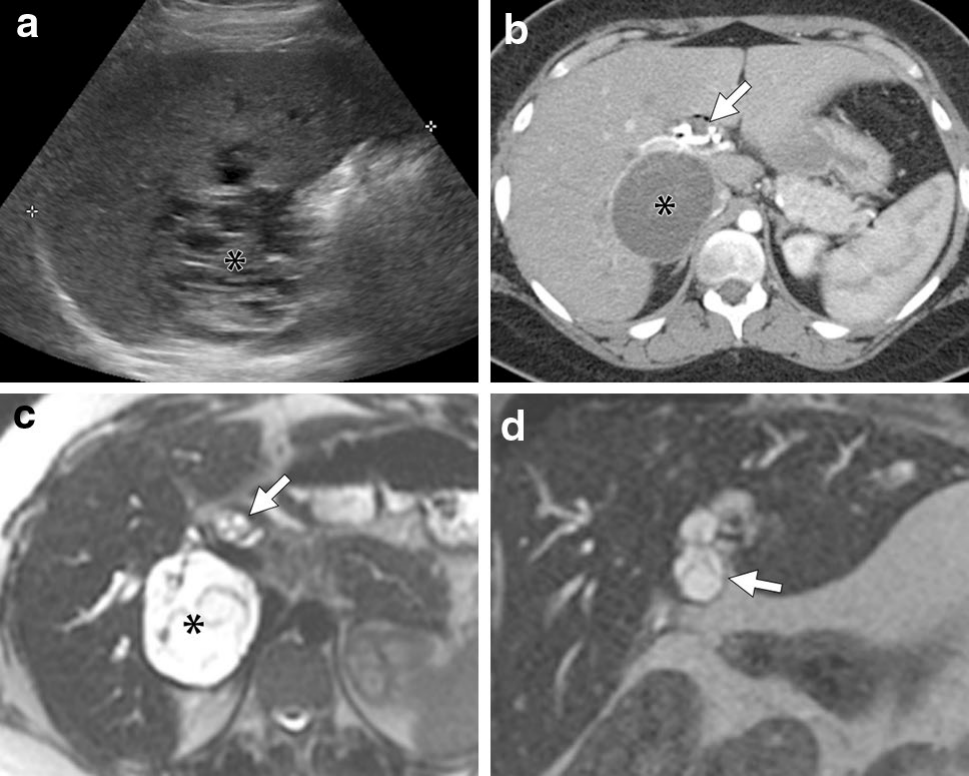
A 22-year-old woman presented with abdominal pain, fever, and jaundice. A 64 mm multilocular cystic mass in the segment VI of the liver was seen on US (a, asterisk), contrast-enhanced CT (b, asterisk), and MRI (c, single shot fast spin echo axial image, asterisk, d. single shot fast spin echo coronal image, asterisk). Arrows indicated the tumoral prolapse to the bile duct. Reader 2 could tell the biliary prolapse on US, CT and MRI, but reader 1 could tell it on US and MRI. Biliary prolapse was better delineated by MRI than CT because, as well as liver tumors, it showed separation (compare arrows in b and c). Abbreviation: GB, gallbladder
